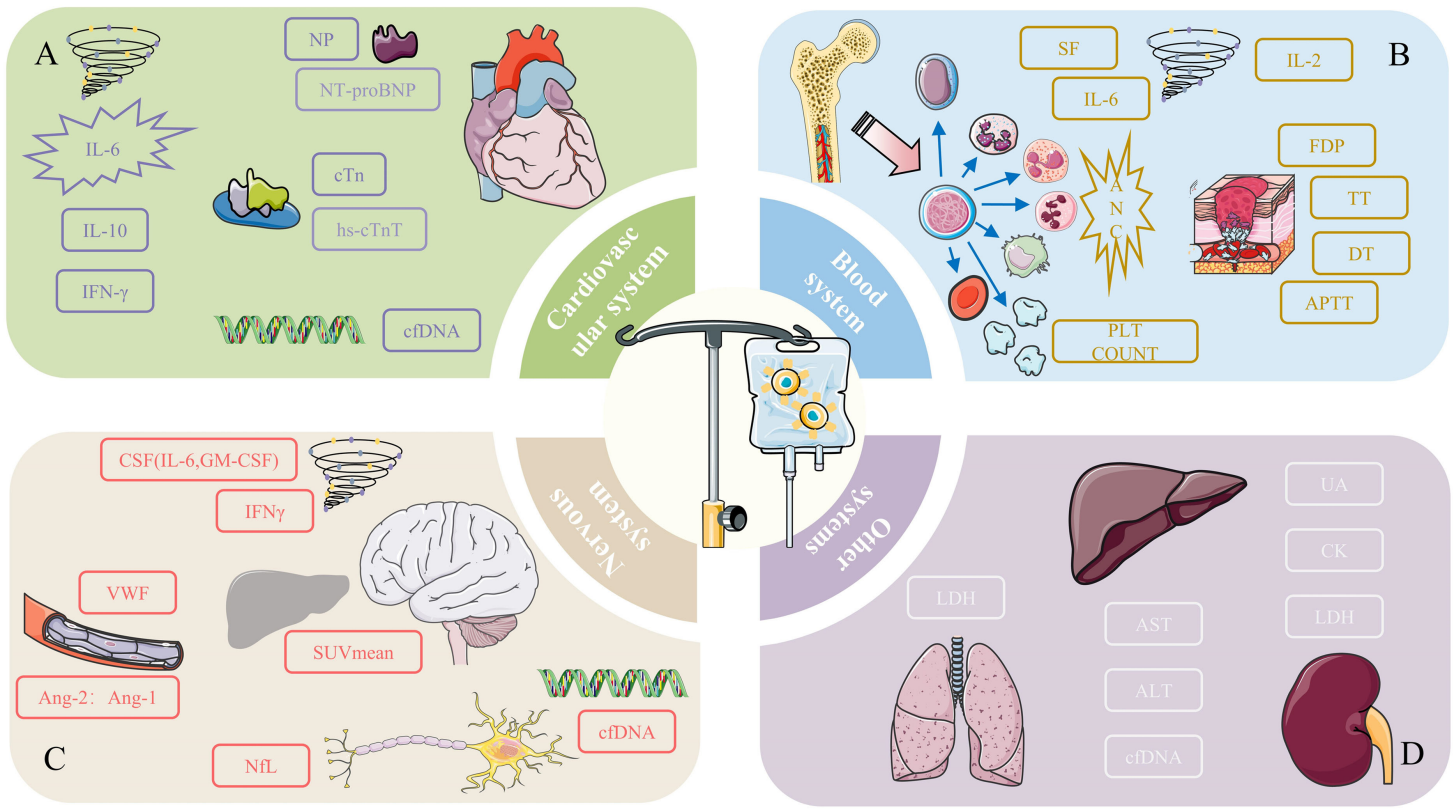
Potential monitoring indicators for preventive toxicity of (A) cardiovascular system, (B) blood system, (C) nervous system, and (D) other systems. *(A) For the cardiovascular system, the designed content in the figure may be the monitoring indicators we can focus on. High concentrations and peak levels of related cytokines (IL-6, IL-10, IFN-γ) are highly associated with adverse cardiovascular events. The peak concentrations of special proteins NT-proBNP and cTn hs-cTnT from the NP family are positively correlated with the severity of cardiotoxicity. Specific cfDNA can be used to predict the occurrence of cardiotoxicity. (B) Blood cell counts are particularly important in the management of hematologic toxicity, especially neutrophil counts. Coagulation-related markers such as FDP, TT, DT, and APTT are used to monitor coagulation disorders after CAR-T therapy. Cytokines (SF, IL-6, IL-2) are also potential monitoring indicators. (C) The cytokines that need special attention in the nervous system are IL-6, GM-CSF, and IFN-γ. Vascular-related active factors such as VWF and the Ang-2: Ang-1 ratio are noteworthy monitoring indicators. NfL, related to axonal injury, is a unique indicator of neurotoxicity. cfDNA can be used to predict the occurrence of toxicity, and the SUVmean of [18F] FDG PET in relevant organs can serve as an indicator of severity. (D) Due to the limited research involving other systems, only potential biomarkers and detection indicators are listed. Their specific roles need further research for confirmation.
